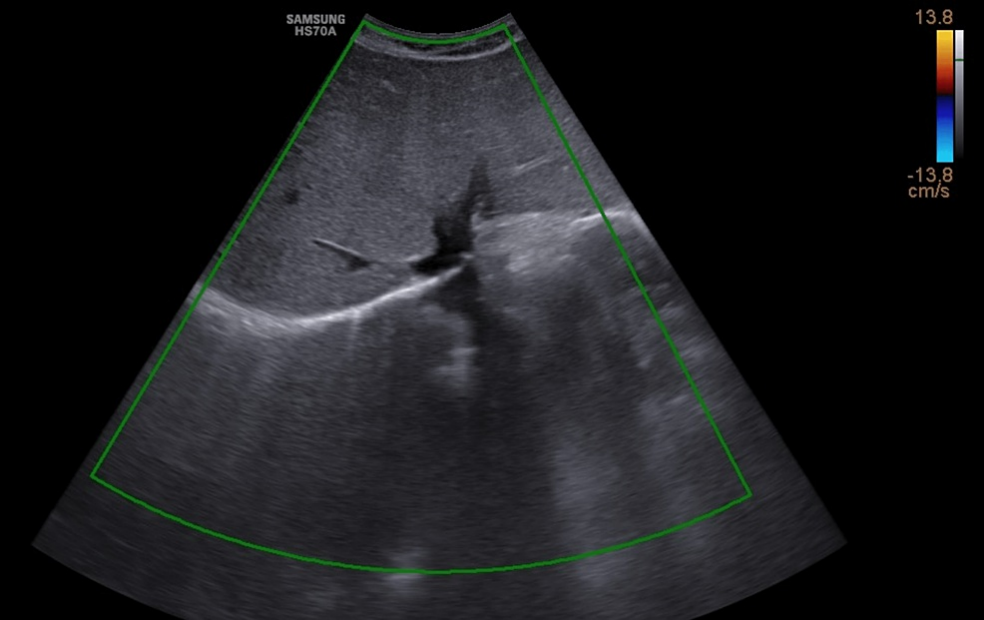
Color Doppler ultrasound showing lack of filling of the hepatic veins draining into the IVC IVC: inferior vena cava
